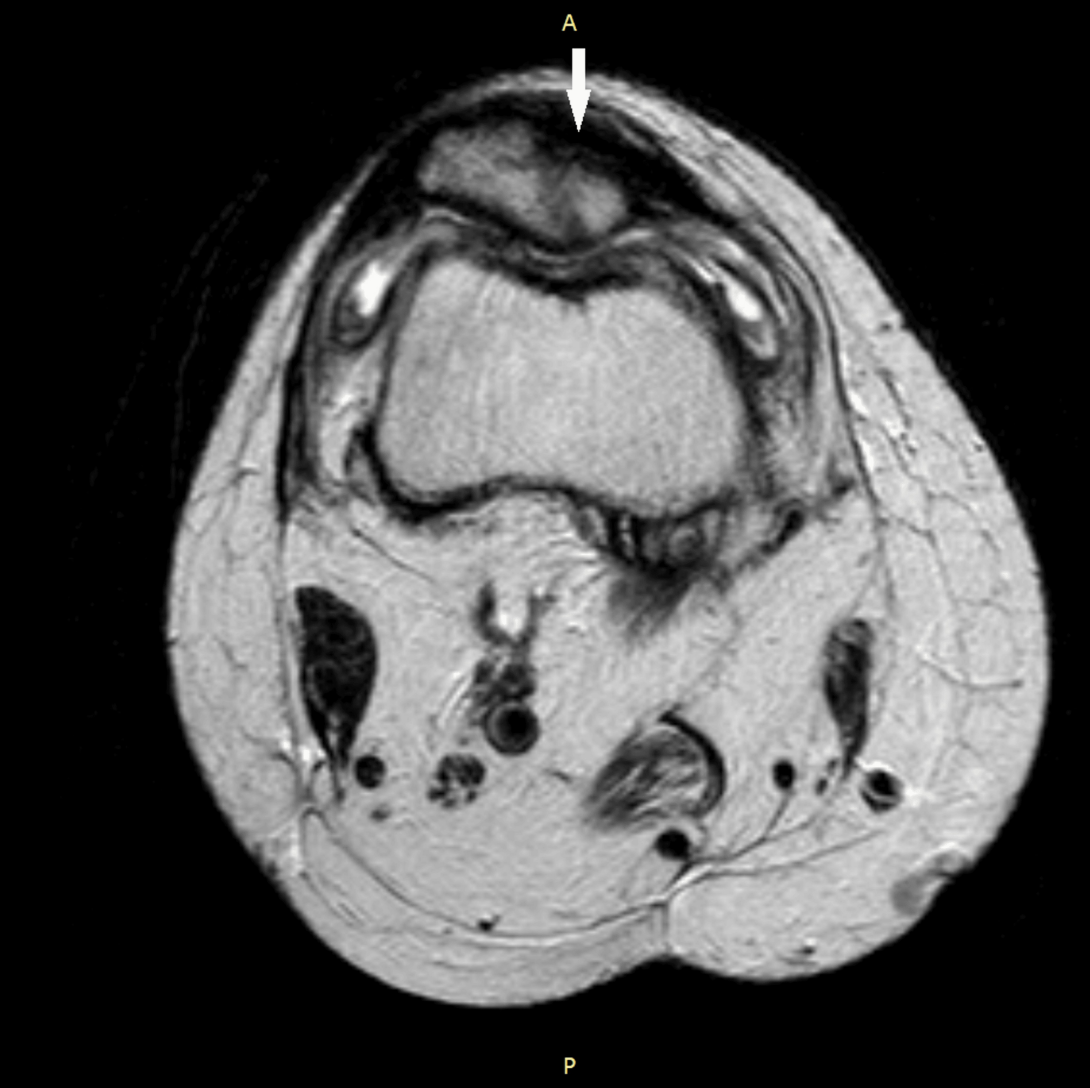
Axial view non-contrast T1-weighted MRI of the patient's right knee demonstrating a large hypodense area involving the inner substance of the patella.
